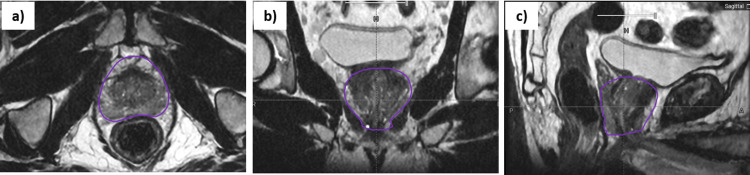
Magnetic resonance images show the enlarged prostate in axial (a), coronal (b), and sagittal (c) views.
